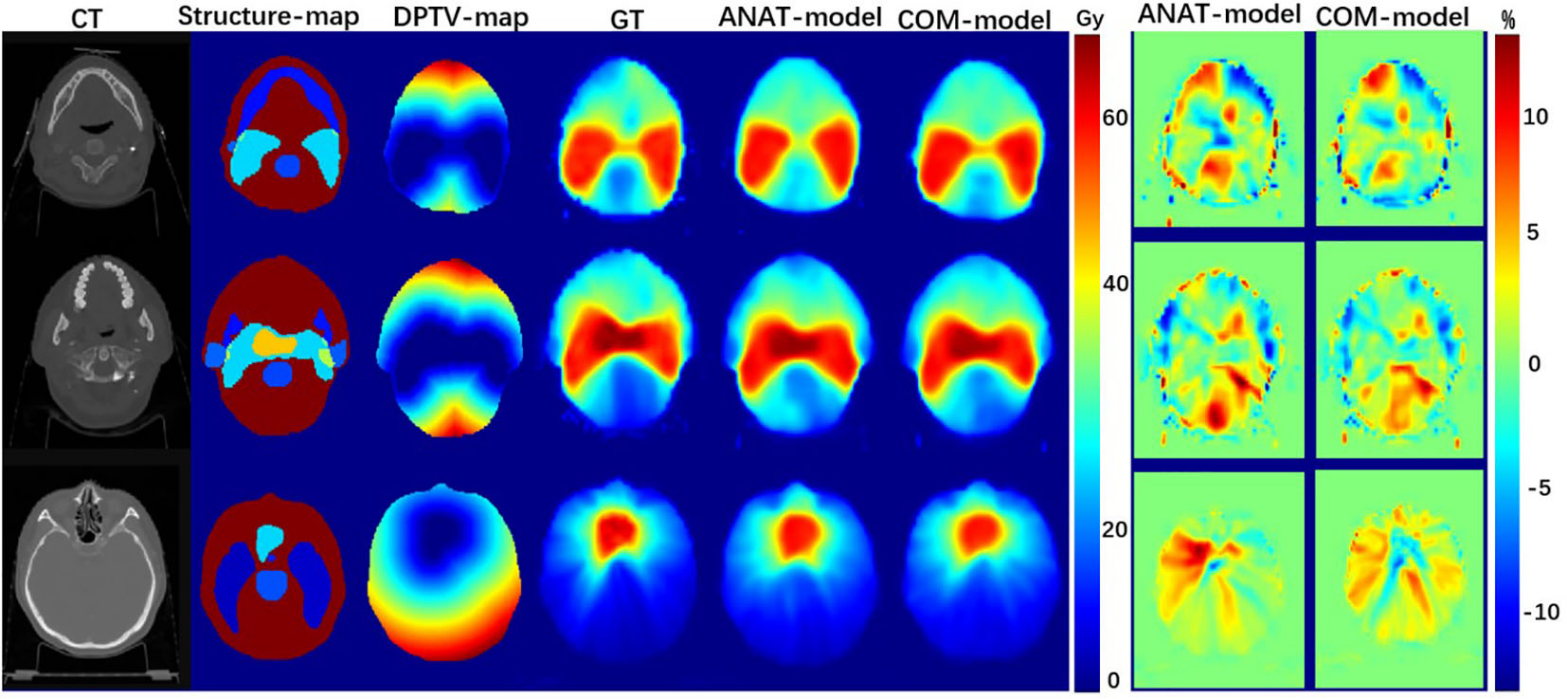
Examples of dose maps predicted by the COM model and ANAT model: the first column, the CT maps as input; the second column, the structure-maps as input; the third column, the DPTV-maps as input; the fourth column, the dose maps of approved treatment plans; the fifth column, the dose maps predicted by ANAT-model; the sixth column, the dose maps predicted by COM-model; the seventh column, the dose-difference maps for ANAT-model; the eighth column, the dose-difference maps for COM-model.
